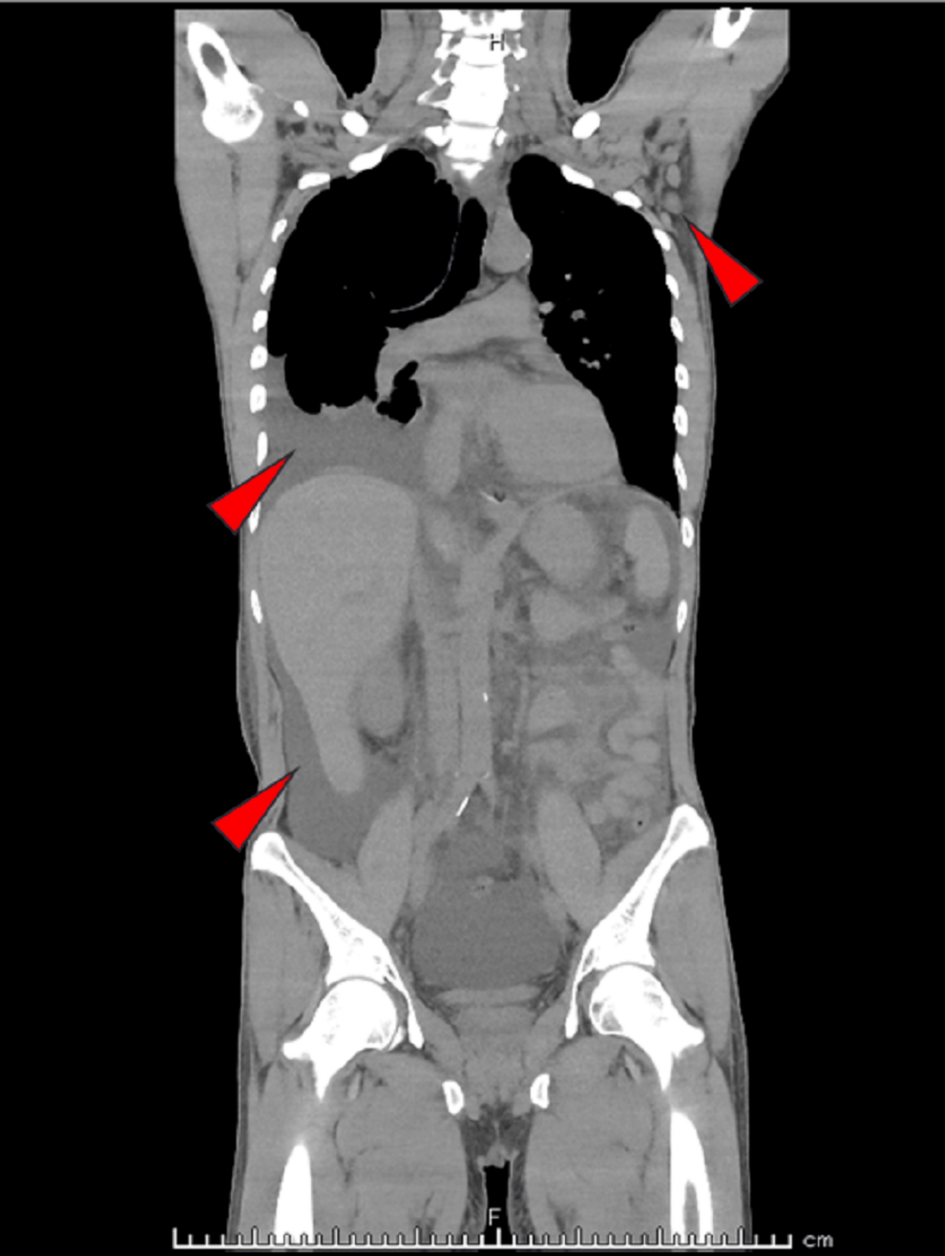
Coronal scan of computed tomography showing pleural effusion, ascites, and mild lymphadenopathy (red arrowheads).
